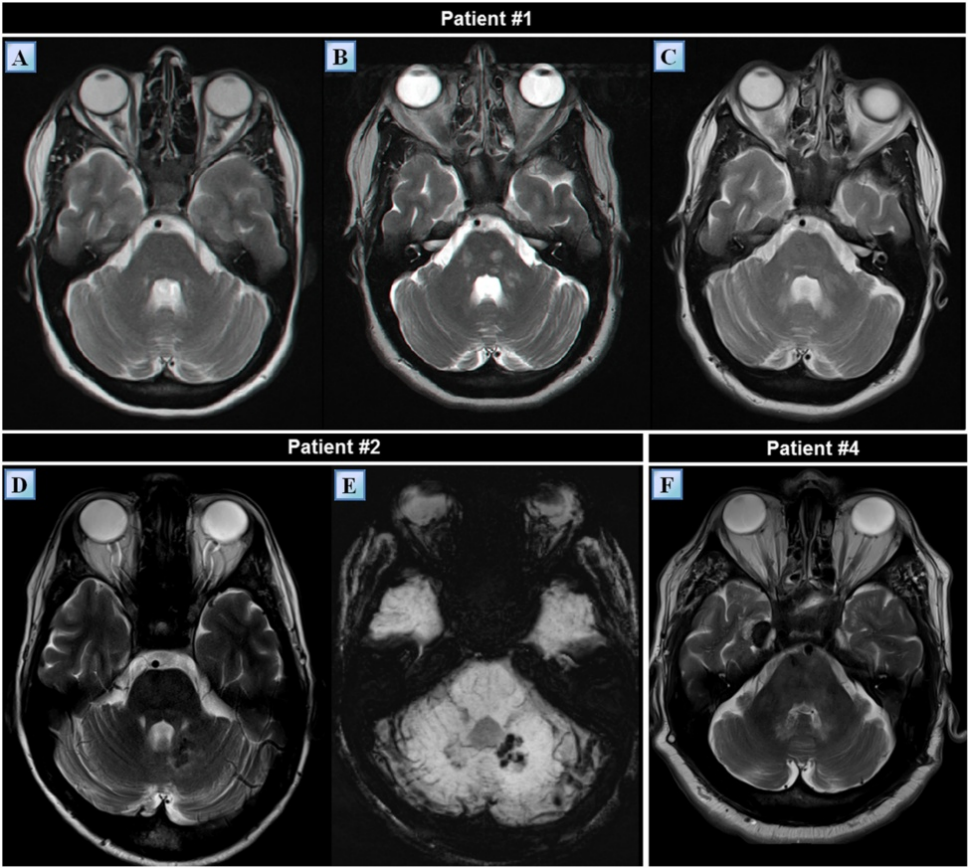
Cerebellar MRI findings of patients #1, #2 and #4 Axial T2 weighted MR images of patient #1 at the level of the cerebellum, middle cerebellar peduncles and pons taken at the time of diagnosis (A) one year following diagnosis (B) and four years following diagnosis (C). Note the bilateral hyperintense signals which emerged one year following diagnosis in the pons and peri-dentate regions as well as in the left middle cerebellar peduncle (B). The signal intensity of these processes seems to have decreased during the following three years (C). (D) An axial T2 weighted MR image of patient #2 at the level of the cerebellum demonstrating a unilateral focus of hypointense signal at the left peri-dentate region. (E) This cluster like lesion is better accentuated in SWI sequence. (F) An axial T2 weighted MR image of patient #4 displaying multiple, bilateral, diffuse foci of hyperintense signals involving the brainstem and peridentate areas. Another abnormal finding, adherent to the dura of Meckel’s cave, exhibits radiological similarity to a meningioma. Note the variable MR manifestations of cerebellar lesions in different ECD patients. ECD, Erdheim Chester disease; MR, magnetic resonance; SWI, susceptibility weighted imaging.
